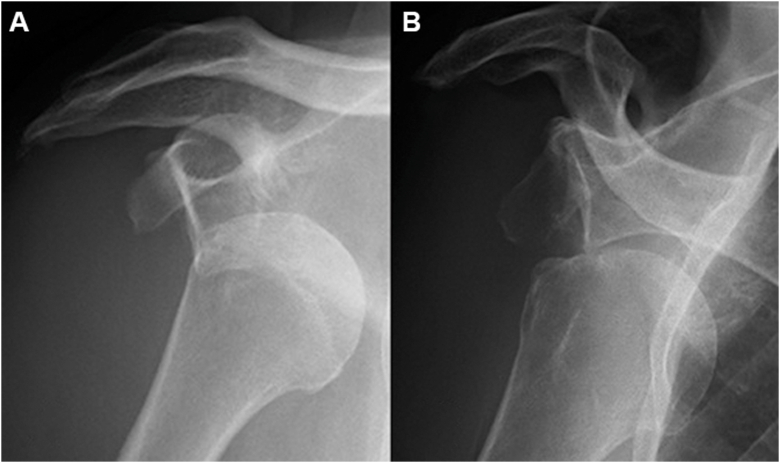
Representative anteroposterior radiographs taken at the time of dislocation showing the position of the greater tuberosity relative to the glenoid. (A) The greater tuberosity is positioned medial to the glenoid. (B) The greater tuberosity is positioned inferior to the glenoid.
